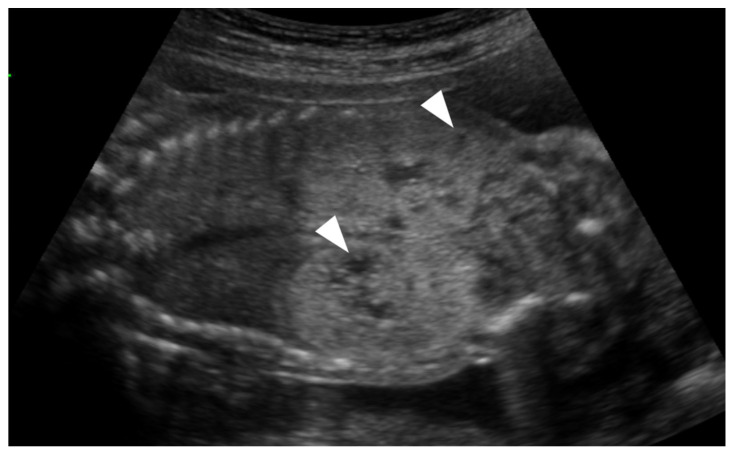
An unusual sonographic pattern of fetal ADPKD (Case 2). Ultrasound image at 22 weeks’ gestation showing markedly enlarged and diffusely hyperechoic kidneys with near-complete loss of corticomedullary differentiation. Small peripheral cysts are also visible (arrowhead). Although diagnosed as ADPKD, the imaging appearance closely resembles the sonographic pattern of ARPKD.
